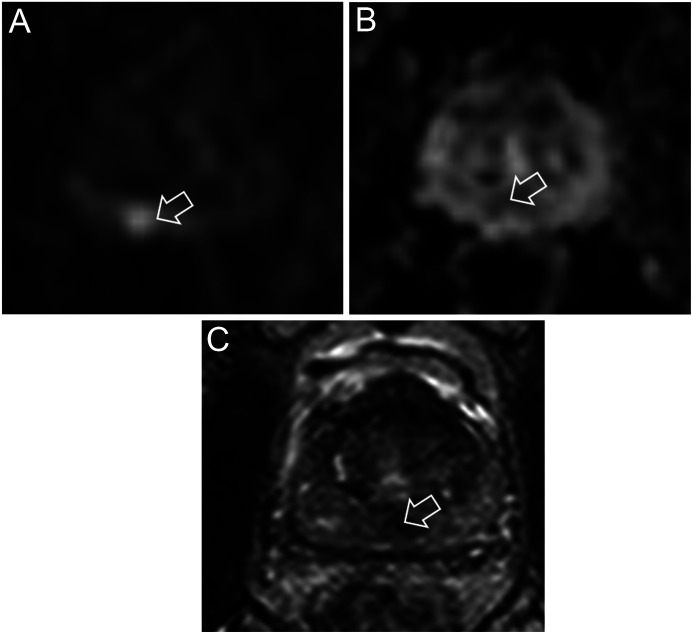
PI-RRADS category 3 based on biparametric MRI, 13 years after RT for prostate adenocarcinoma Gleason score 7, in 77-year-old patient with increased PSA 2.09 ng/mL The lesion detected as hyperintense on DWI at high *b* values (arrow in A) and moderately hypointense on ADC map (arrow in B) and hypointense inhomogeneous on axial T2W imaging (arrow in C) occurs at the site of the tumor of origin in the base in the right median segment of the peripheral zone.
